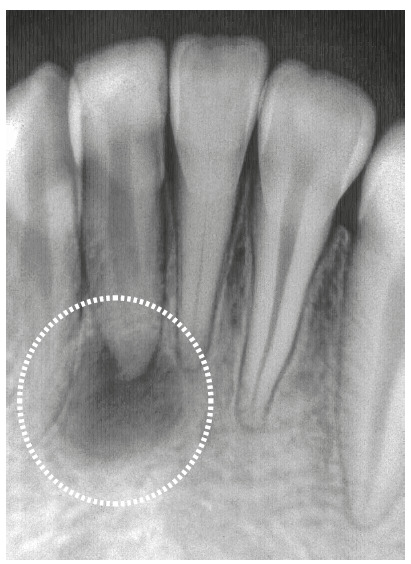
Chronic periapical lesion compatible with periapical granuloma associated with aseptic pulp necrosis caused by products of tissue necrosis.
